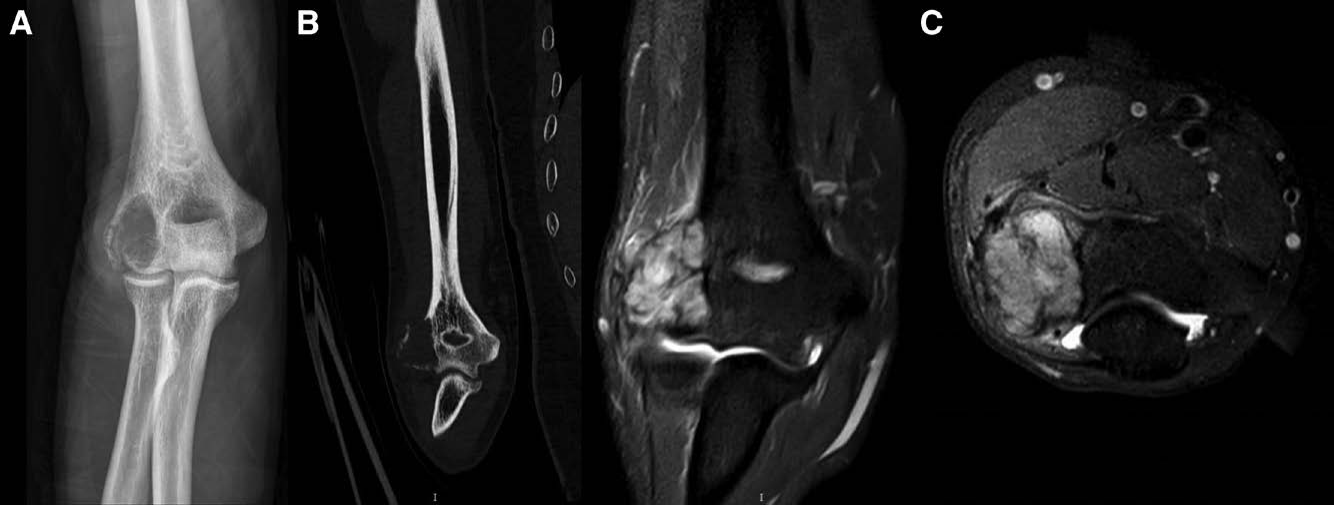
Imaging examination before operation. (A) X-ray, (B) CT, and (C) MRI.
